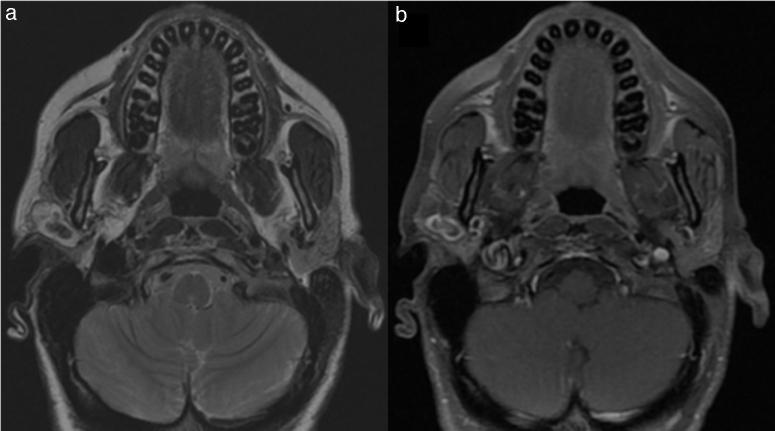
(a) T2-weighted axial sequence shows heterogeneous hyper signal intensity, due to the presence of areas of marked low signal intensity. (b) T1-weighted FAT-SAT axial sequence after medium contrast intake shows an irregular enhancement, since the medium contrast uptake was absent in the central and in the cystic areas, but it was higher in the solid peripheral component of the tumor.
